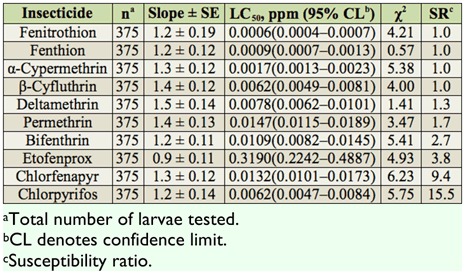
Summary of toxicities of 10 insecticides to third instars of the BS-CT strain of *Culex tritaeniorhynchus* based on the 24 hr exposure contact mortality bioassays.
